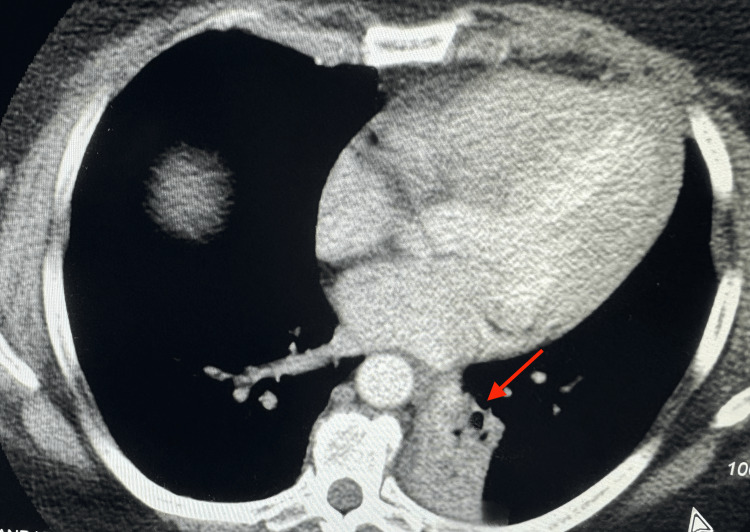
Axial CT chest displaying atelectasis of the left lower lung from the endobronchial lesion (arrow)
